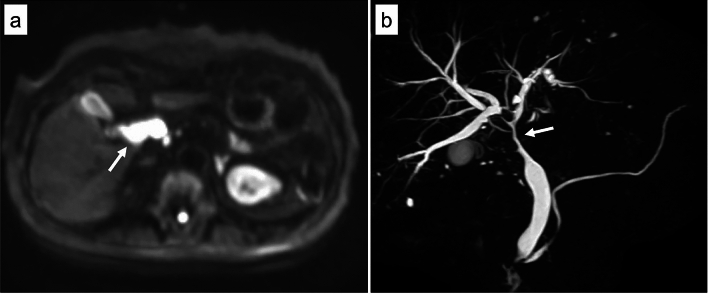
Magnetic resonance imaging. a The thickened wall of the perihilar bile duct and adjacent mass shows a hyperintense signal on diffusion-weighted imaging (arrow). b Magnetic resonance cholangiopancreatography reveals stricture of the bile duct from the hepatic hilum to the vicinity of the confluence of the cystic duct (arrow), and the upstream bile duct is slightly dilated
